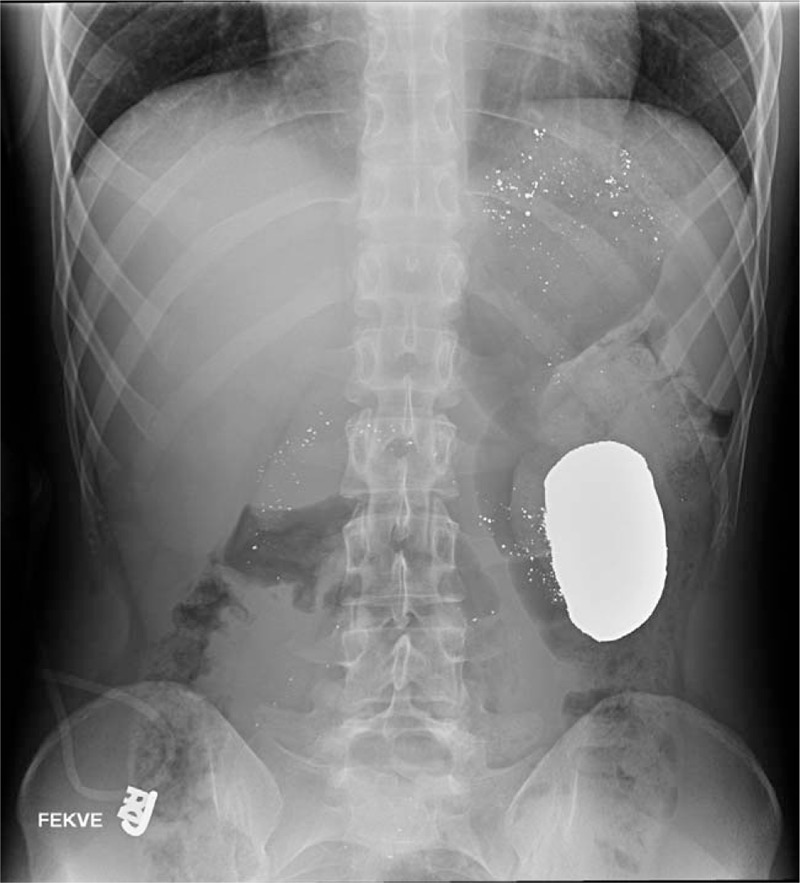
Metallic density in the area of the stomach. The mercury was ingested 3 hours before radiography. Anteroposterior radiography in supine position.
